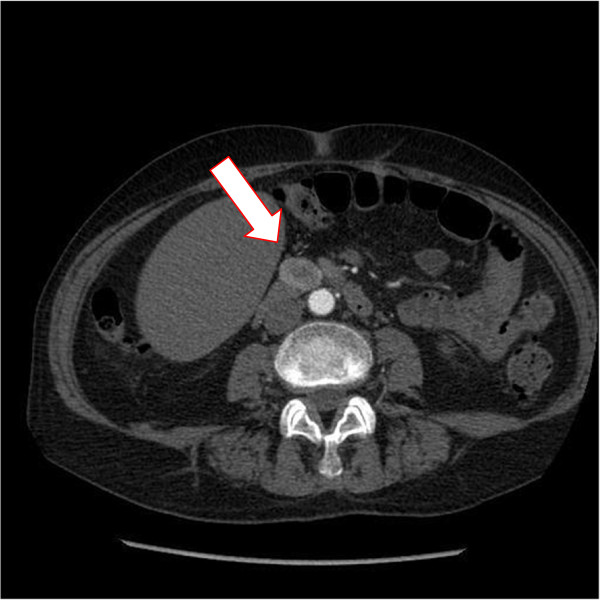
Abdominal CT scan showing a 2-cm, contrast-enhanced mass (arrow) in the uncinate process of the pancreas.
